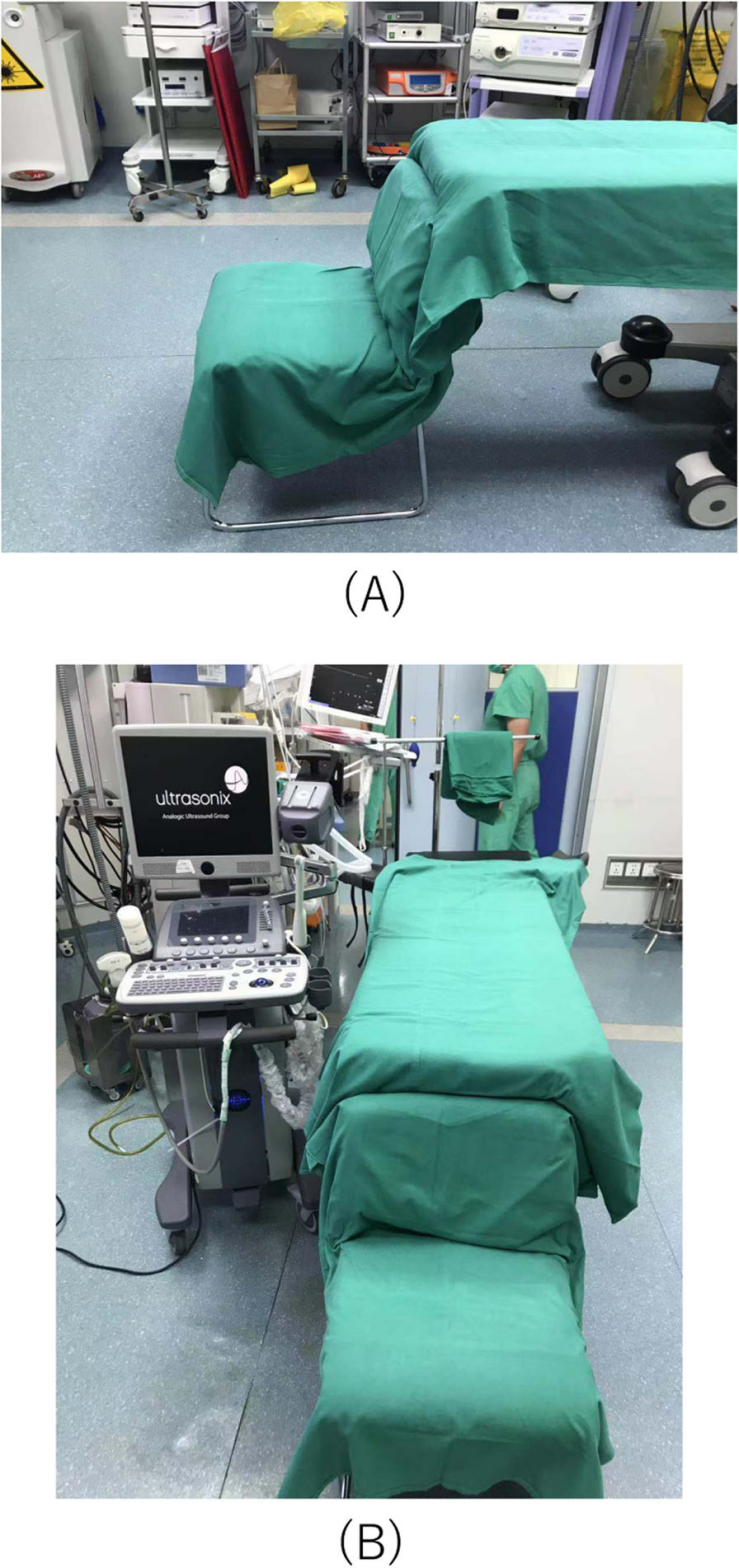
a Chair with back was put at the rear end of the operating table. b The Ultrasonic instrument was placed on the left side of the chair
